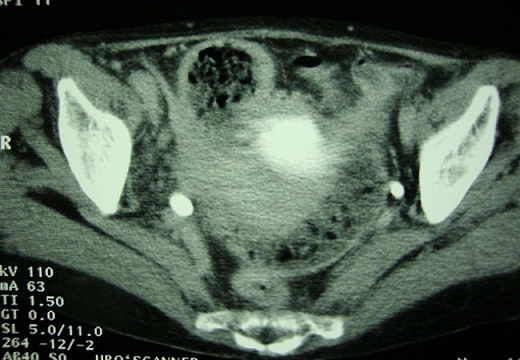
CT scan: small bowel segment adherent to the bladder with feces sign
